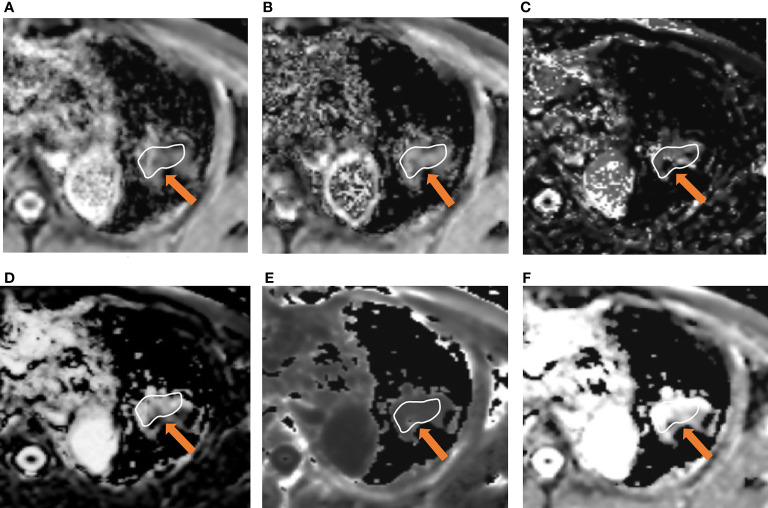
A 66-year-old male was diagnosed with lung adenocarcinoma in the left upper lobe. Parameter map of ADC, D, D*, f, Kapp, and Dapp calculated from multiple b-value DWI data (A–F). The calculated mean values of ADC, D, D*, f, Kapp, and Dapp were 0.84×10^-3^ mm^2^/s, 0.65×10^-3^mm^2^/s, 35.23×10^-3^ mm^2^/s, 32.31%, 1.01, and 2.21×10^-3^ mm^2^/s, respectively.
